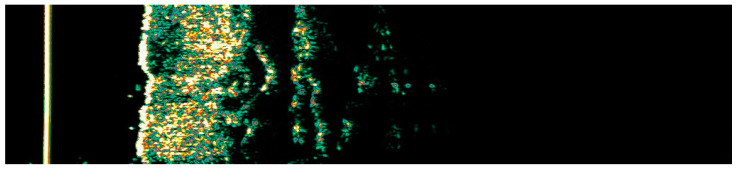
Ultrasonographic image of BCC on the neck.
